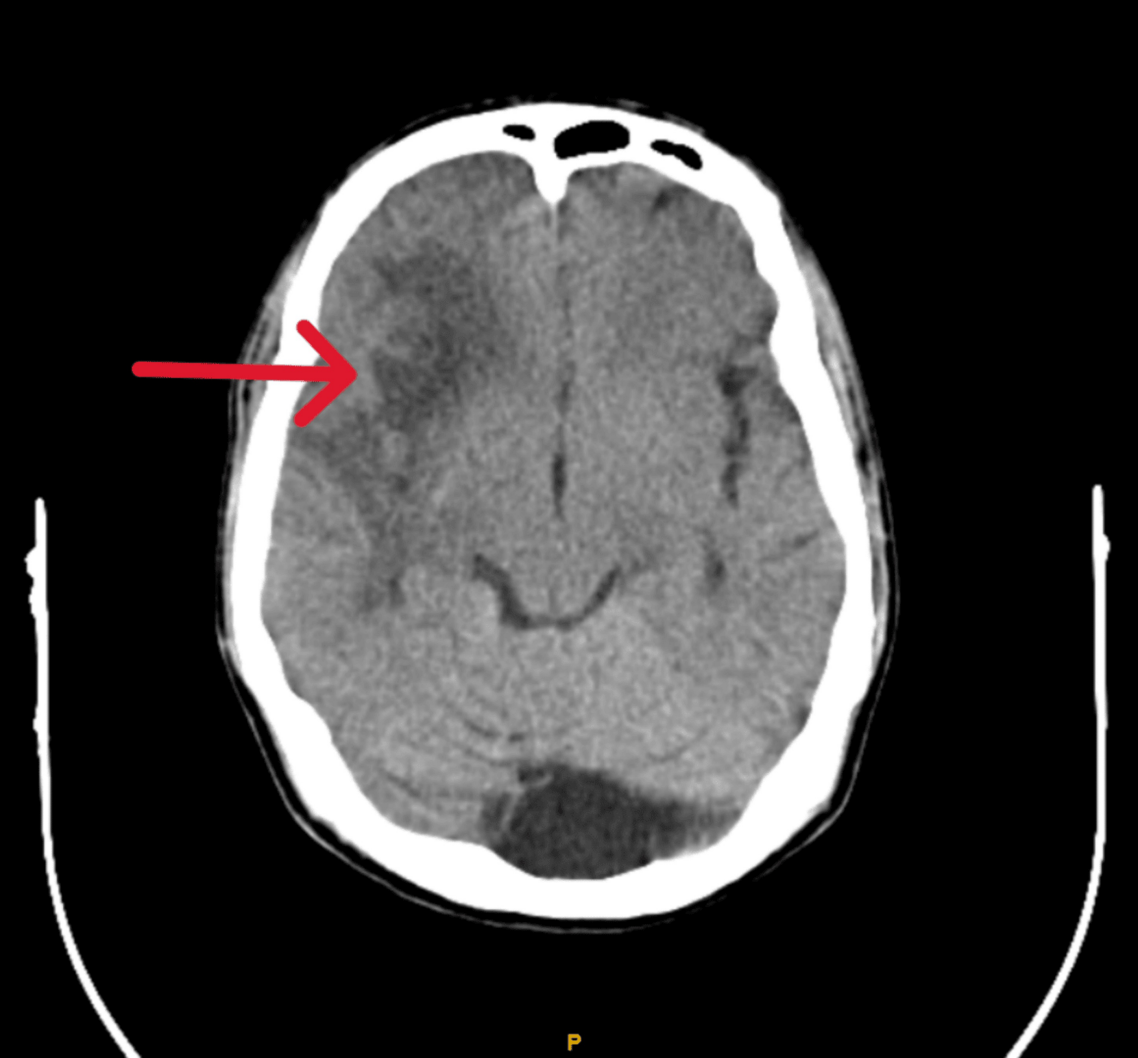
CT scan of the brain showing sub-acute infarct of the right frontal-parietal-temporal regions involving the right ganglion-capsular regions and right corona radiata (red arrow). CT: computed tomography
